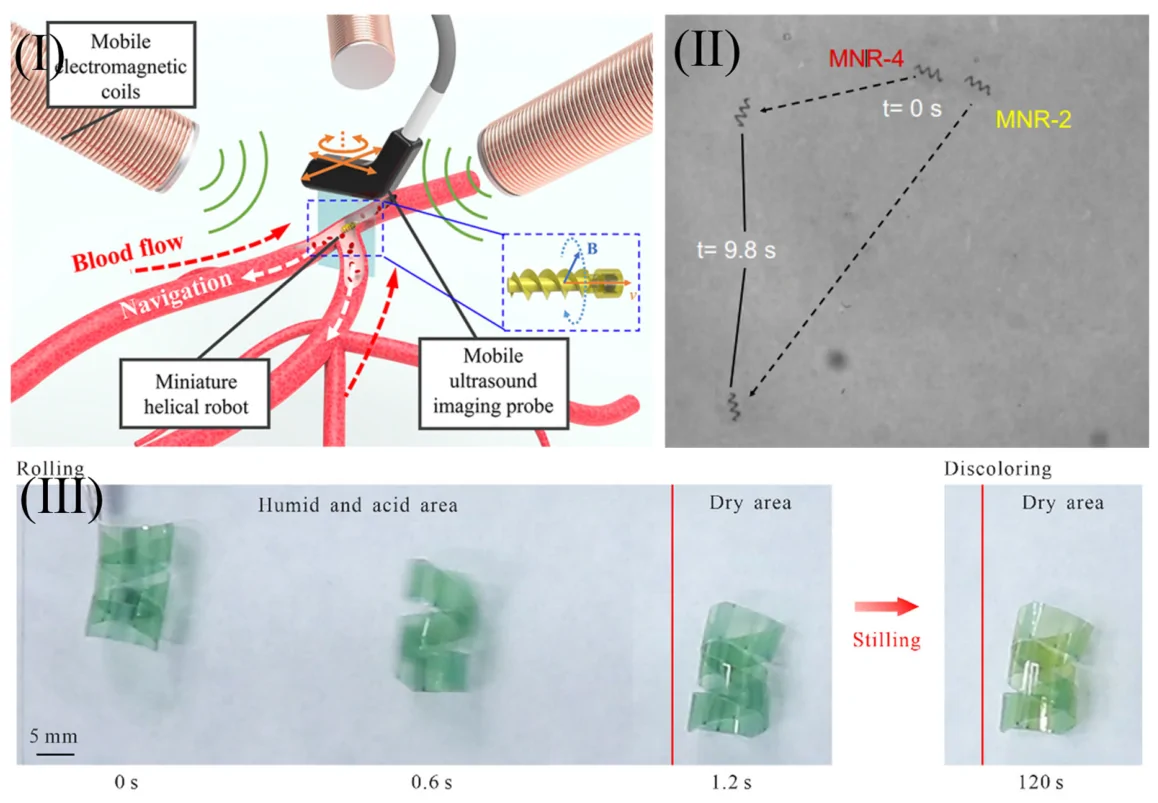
Helical microrobots: (I) An ultrasound Doppler imaging-guided helical microrobot for targeted thrombolysis. Reprinted with permission from Ref. [81]. Copyright 2022 American Chemical Society. (II) A comprehensive fluid–solid interaction dynamic model that enables precise navigation for general helical micro-/nanorobots. Reproduced from Ref. [82] under the terms of the Creative Commons Attribution-NonCommercial-NoDerivatives 4.0 International License (CC BY-NC-ND 4.0). (III) A double-helical soft robot driven by constant humidity that achieves ultra-fast rolling locomotion through alternating deformation of two helices. Reproduced from Ref. [83] under the terms of the Creative Commons Attribution 4.0 International License (CC BY 4.0).
